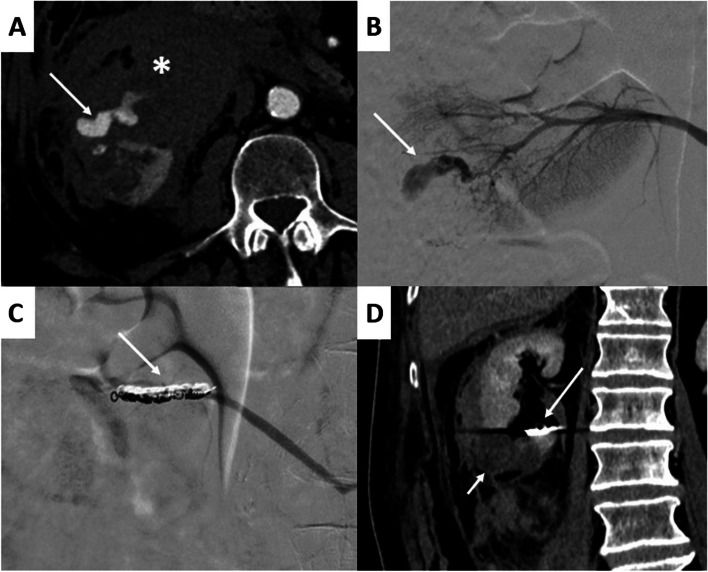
A 63-year-old man who underwent a renal biopsy 4 h earlier. The patient presented with a sudden drop in hemoglobin levels accompanied by pallor. A The axial view of an arterial-phase contrast-enhanced abdominal CT scan showed a large right retroperitoneal hematoma (*) along with active renal bleeding (arrow) from the lower pole of the right kidney. B Angiography of the right lower polar artery confirmed active bleeding (arrow). C Follow-up angiography after placement of two microcoils (arrow) shows cessation of the active bleeding. D Contrast-enhanced CT scan at portal phase in coronal section revealed limited necrosis of the lower pole (short arrow) with coil artifacts (long arrow)
